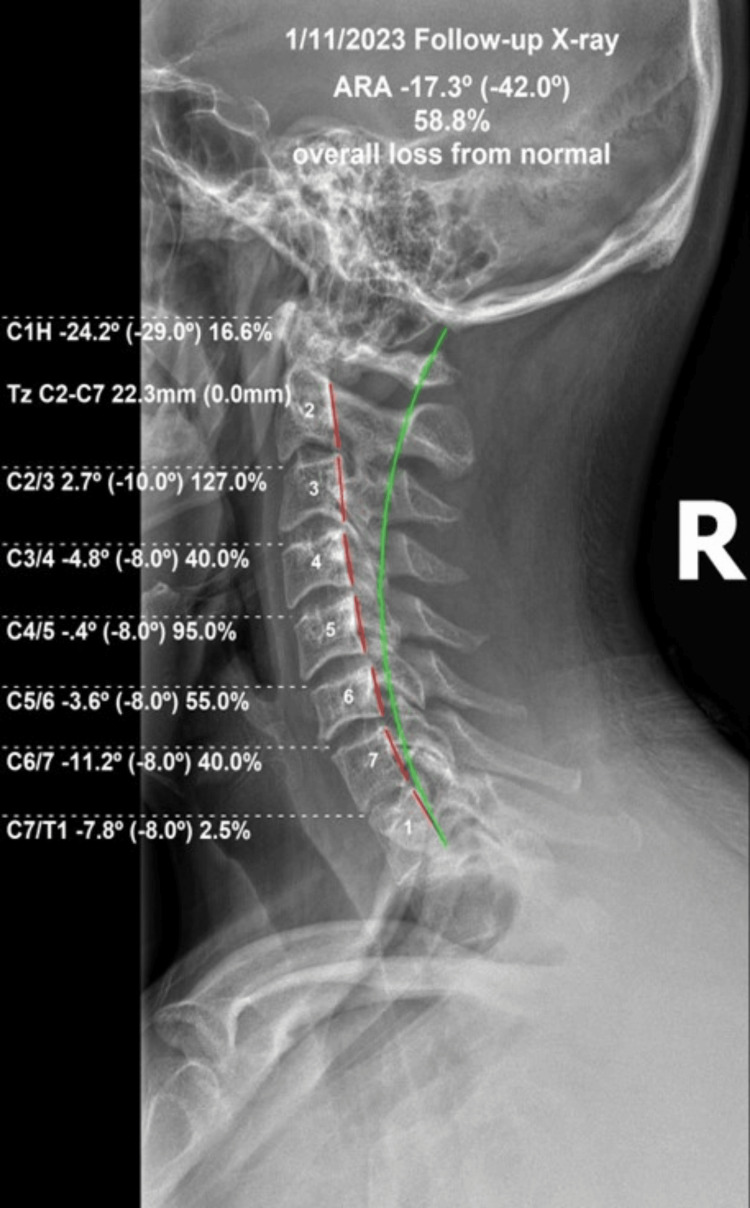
Post-treatment re-examination lateral cervical radiograph following 24 in-office treatments using CBP® protocol. The cervical lordosis improved to -17.3° (vs. -1.3° initially), the atlas plane line improved to -24.2° (vs. -10.9° initially), and the anterior head translation reduced to 22.3 mm (vs. 35 mm initially). The kyphotic C2-C3 segment improved to +2.7° (vs. +9.7° initially). CBP®: Chiropractic BioPhysics®.
